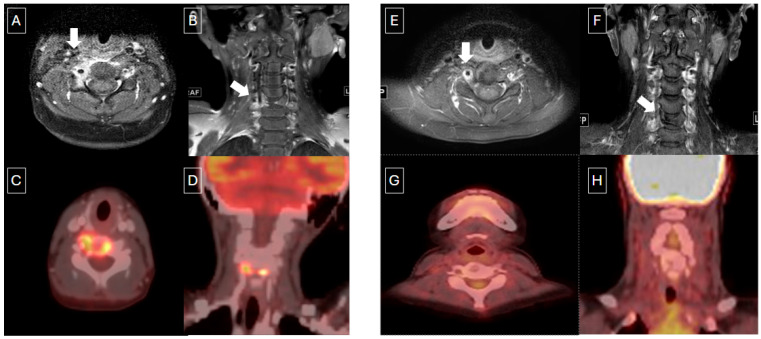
Nine-year-old male with multifocal bone LCH. (A,B) Axial and coronal T1 post-contrast MR images show vertebra plana and soft tissue enhancement (white arrows) with corresponding increased metabolic activity on PET/CT (C,D). End-of-therapy assessment on MR (E,F) showed some enhancement in the soft tissues and persistent vertebra plana. However, post-treatment FDG PET/CT (G,H) demonstrated complete metabolic response.
